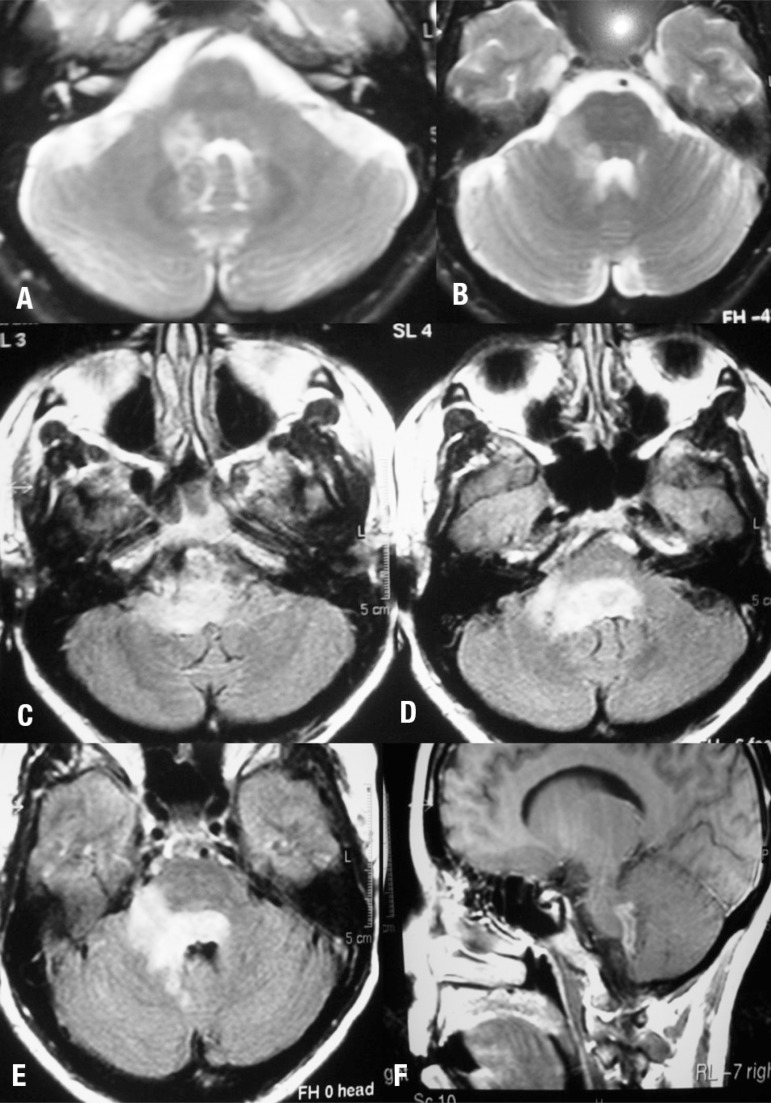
MRI- T2-weighted images [A, B], FLAIR [C, D, and E], T1 with gadolinium
